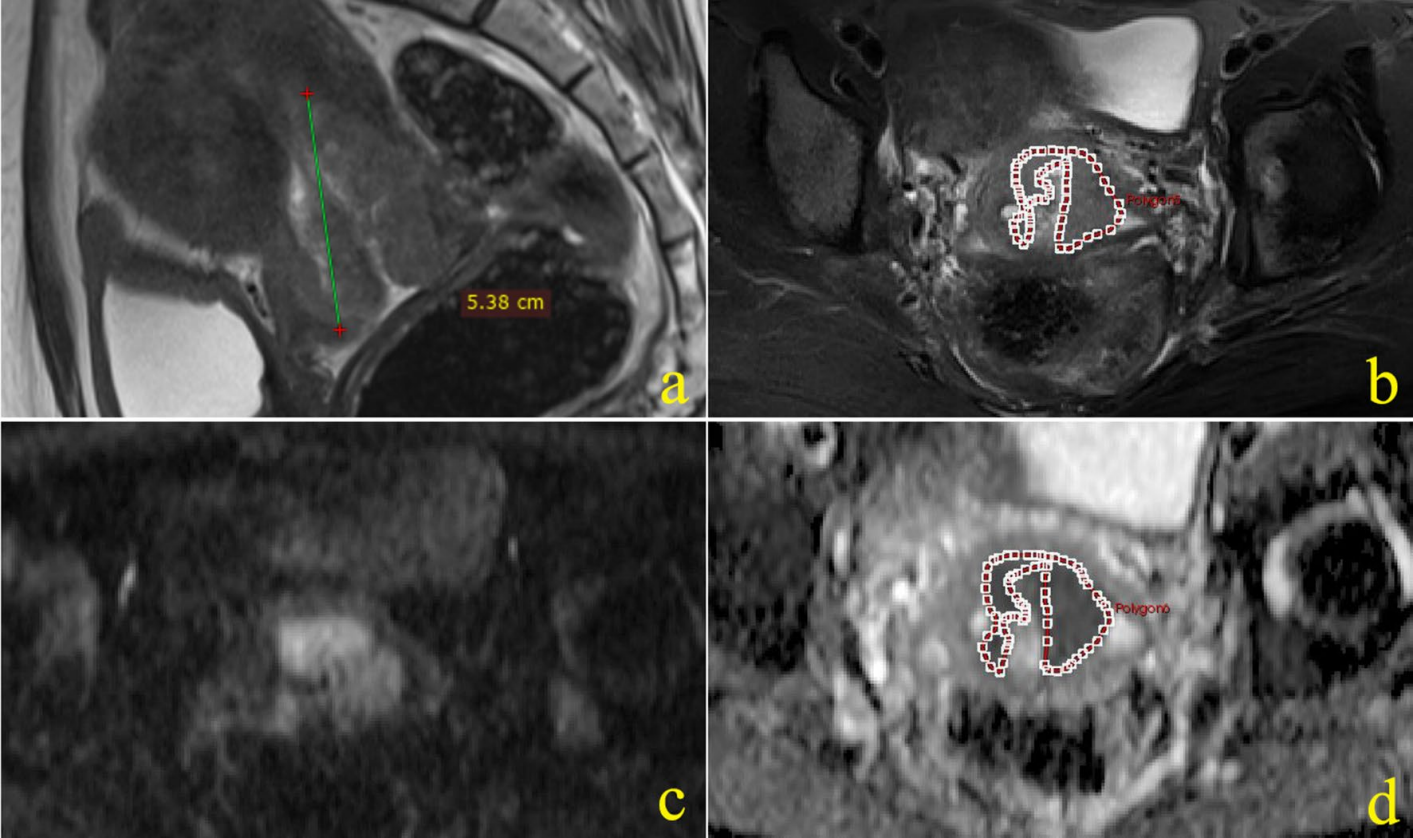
Images of a 45-year-old cervical cancer patient, (a) sagittal T2-weighted image of the tumor, the maximum diameter of tumor showed at sagittal image was 53.8 mm; b–d showed manually drew ROI at the axial largest slice of the tumor on different sequences, (b) axial fat suppressed T2-weighted FSE sequence; c axial DWI (*b* = 1000 s/mm^2^) sequence; d ROI of tumor on the corresponding slice of ADC map. FSE, fast spin-echo; DWI, diffusion-weighted imaging; ADC, apparent diffusion coefficient; ROI, regions of interest
